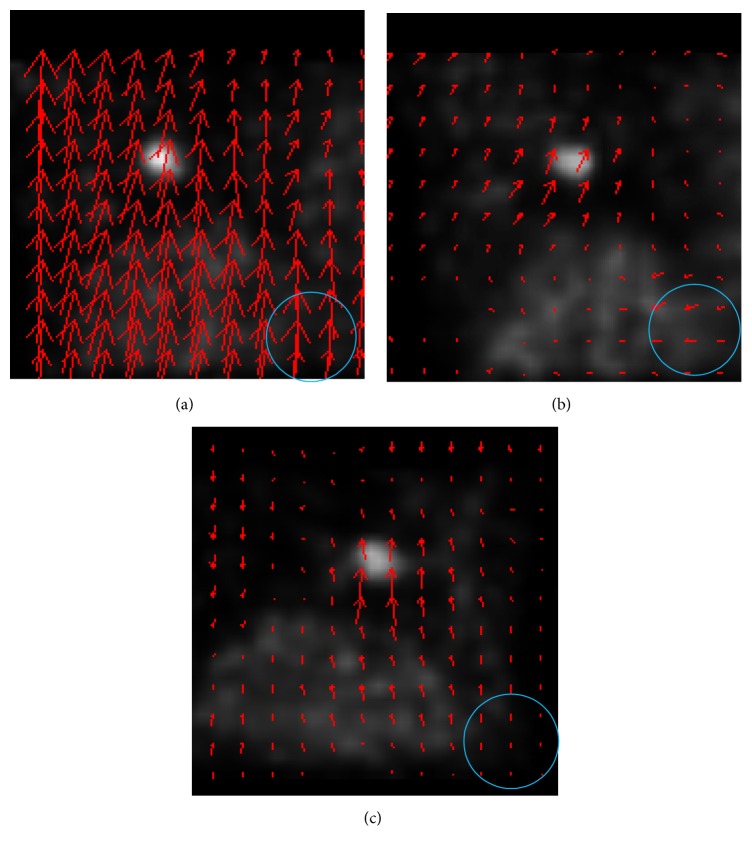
MVF obtained using only exhalation images. (a) From CT images. (b) Previous RSM. (c) Proposed RSM.
